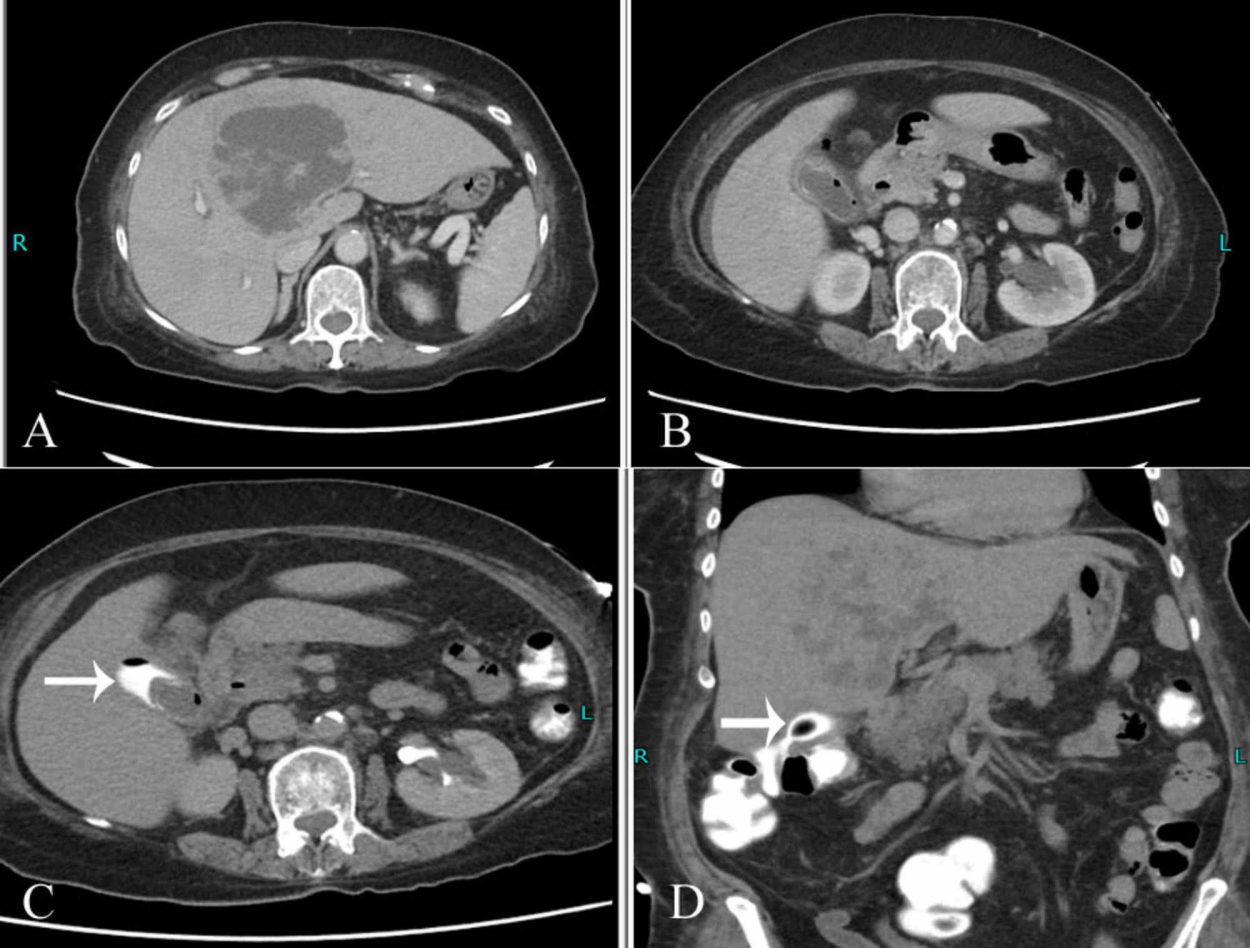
Computed tomography images A) Large necrotic liver abscess noted in segment IV. B) Specks of air seen within the gallbladder lumen. C & D) After rectal contrast administration, opacification of the gallbladder was noted (arrow) confirming the suspicion of cholecystocolonic fistula.
